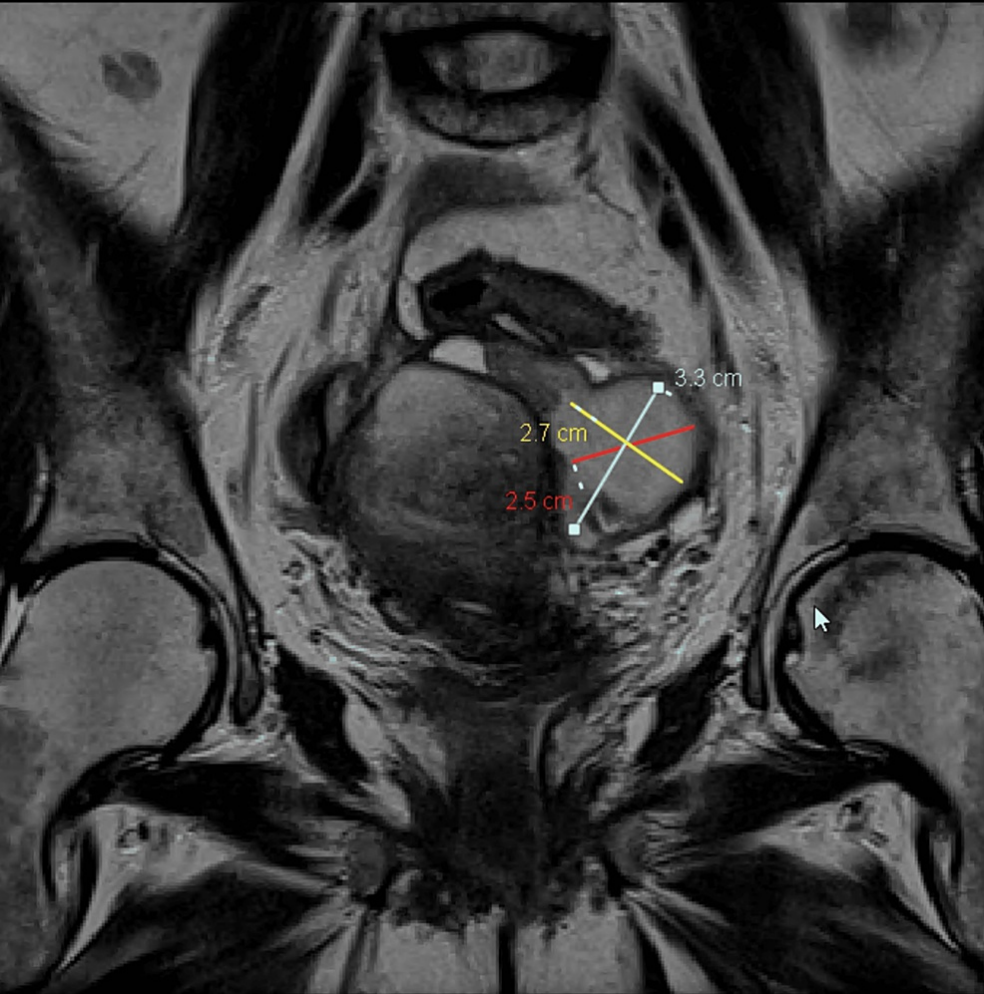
MRI of the pelvis showing the left ovarian collection
